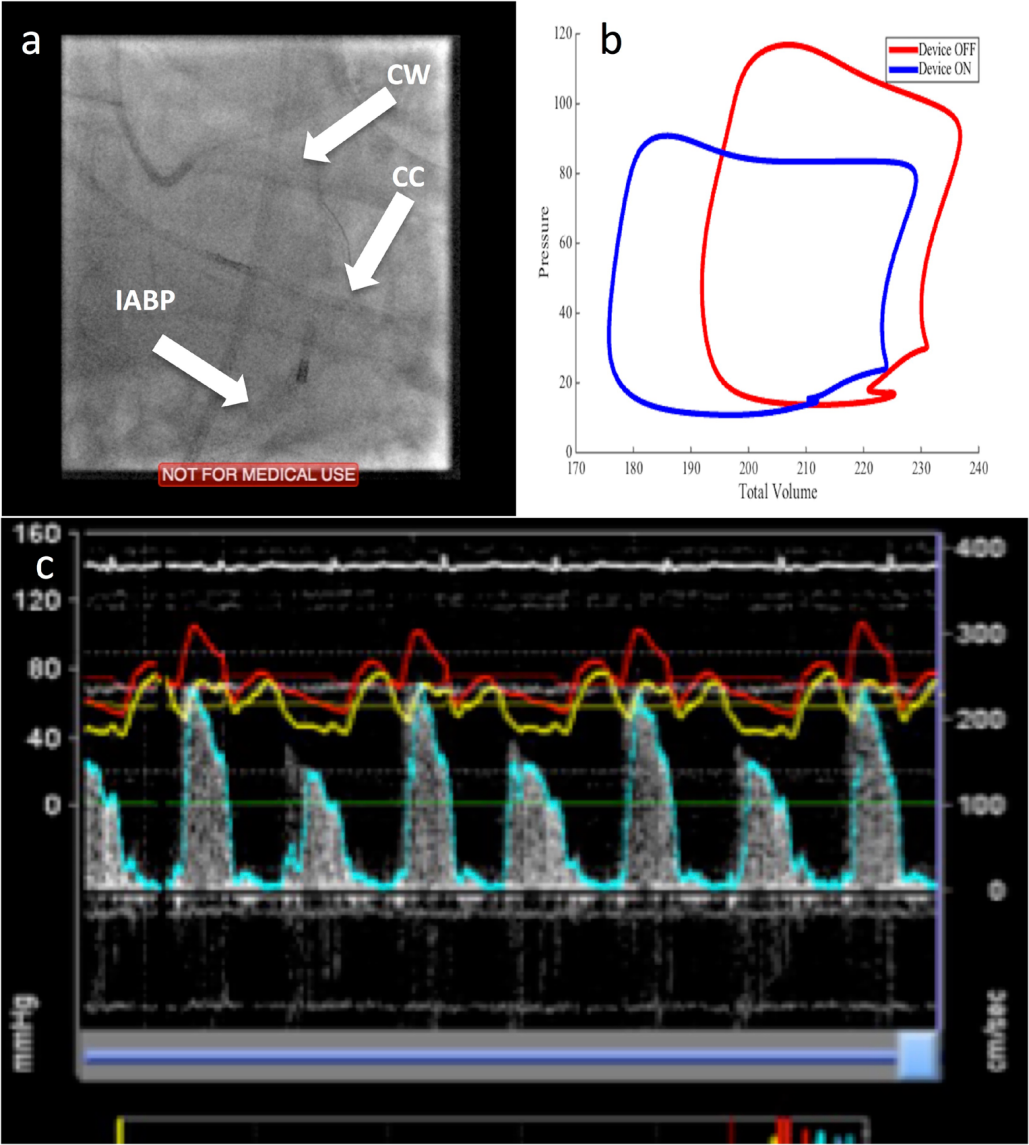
Study haemodynamic data acquisition. a Fluoroscopic placement of IABP catheter in the descending aorta, with ComboWire (CW) visualised in the left anterior descending artery and a PV loop catheter (CC) in the LV. b Representative PV loop obtained with and without IABC support. c Representative coronary data acquisition with the ComboWire and IABC support at 1:2 (red: aortic pressure, yellow: distal coronary pressure, blue: flow velocity (Doppler) trace)
